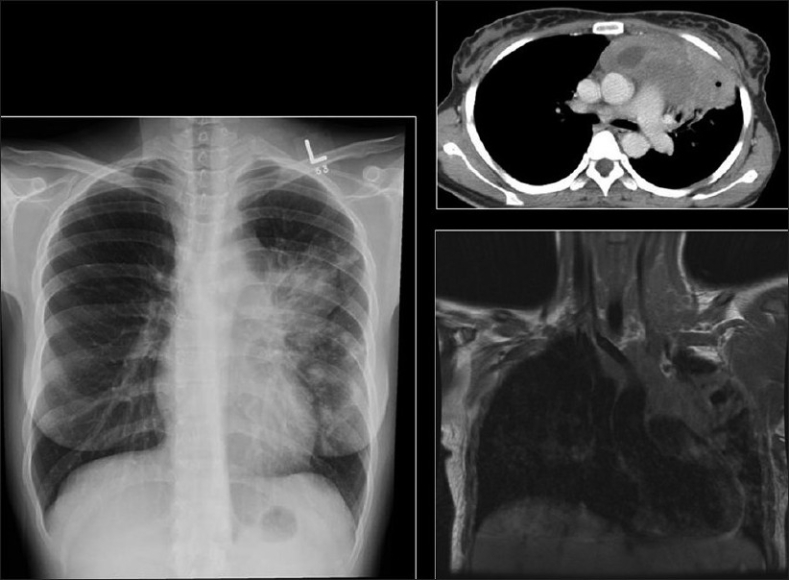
NHL in a 23-year-old human immunodeficiency virus female. The chest radiograph shows multiple well-defined lung nodules within the left lung associated with mediastinal lymphadenopathy. Lymphadenopathy is a less common feature in acquired immunodeficiency disease-related NHL and nodes are rarely significant according to size criteria unlike as in the case shown here, where there is significant lymphadenopathy as confirmed by computed tomography (right upper frame). Magnetic resonance imaging is the imaging of choice to detect vascular encasement
